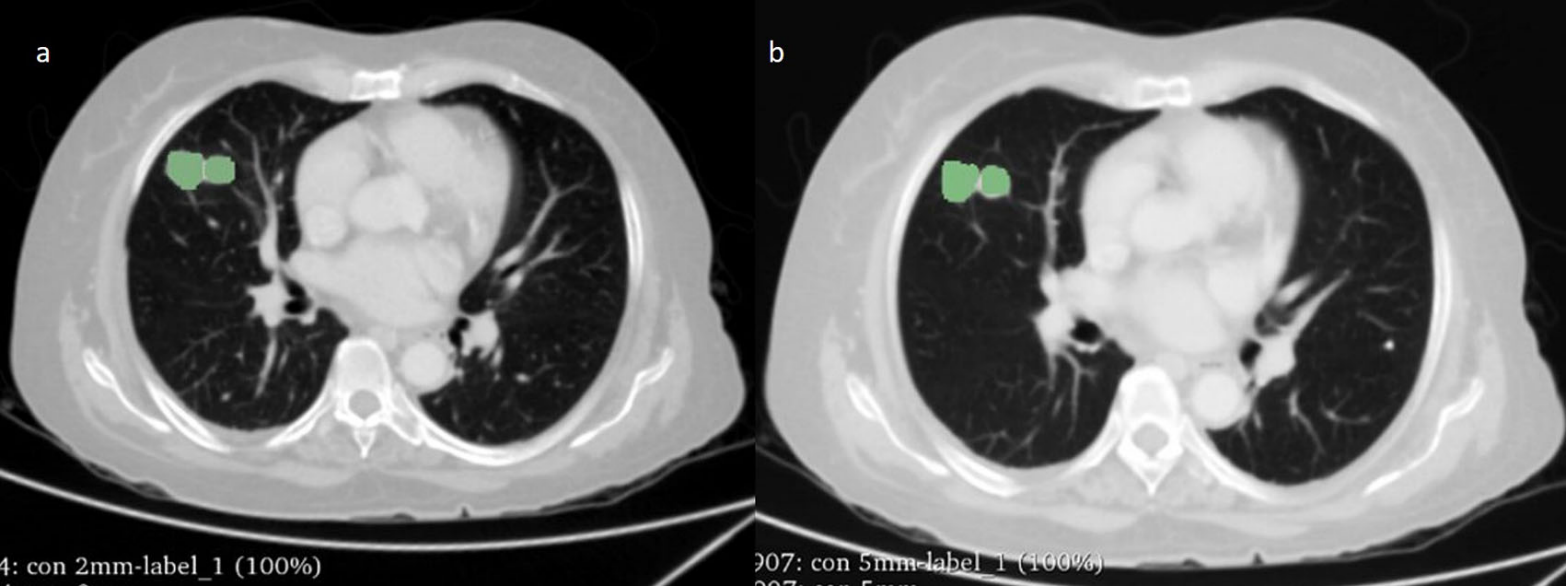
An axial CT image of a 52-year-old male with adenocarcinoma showing manual segmentation of tumour using 3D Slicer at (a) 2mm and (b) 5mm slice thickness.
